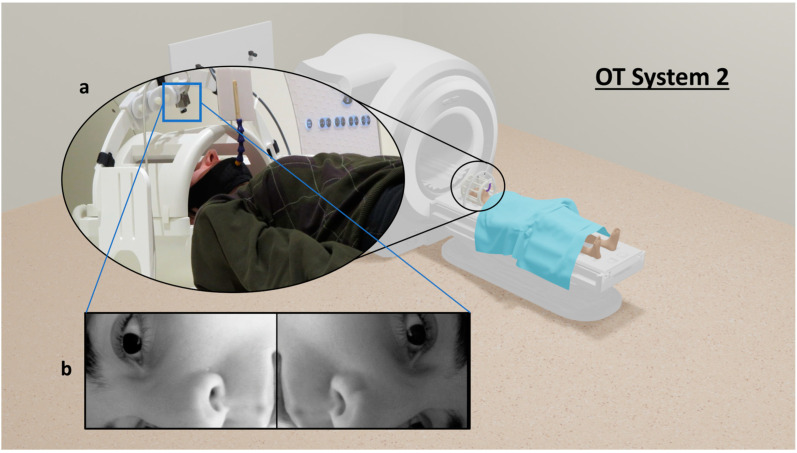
OT System 2. (a) Two in-bore, MRI-compatible cameras are positioned on an arch above the head coil. (b) The cameras point through the rungs of the head coil to view the left and right side of the face. OT = Optical Tracking.
